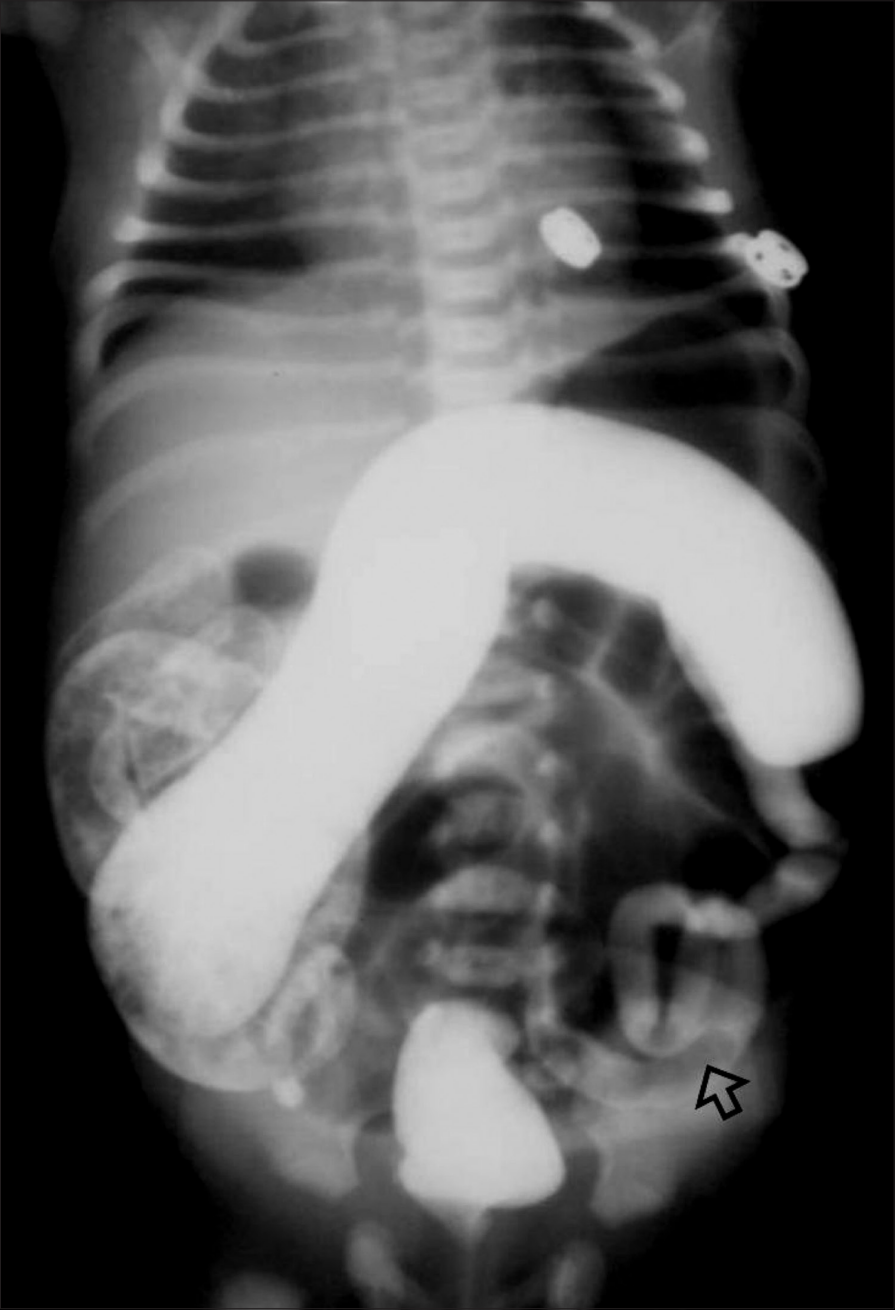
Small left colon syndrome: Frontal radiograph of the abdomen as part of a contrast enema examination using iodinated contrast (gastrografin) shows a narrow caliber of the left colon, with an abrupt transition at the splenic flexure. Multiple filling defects in the left colon are due to meconium plugs (arrow). Also note the rectum is quite distensible
